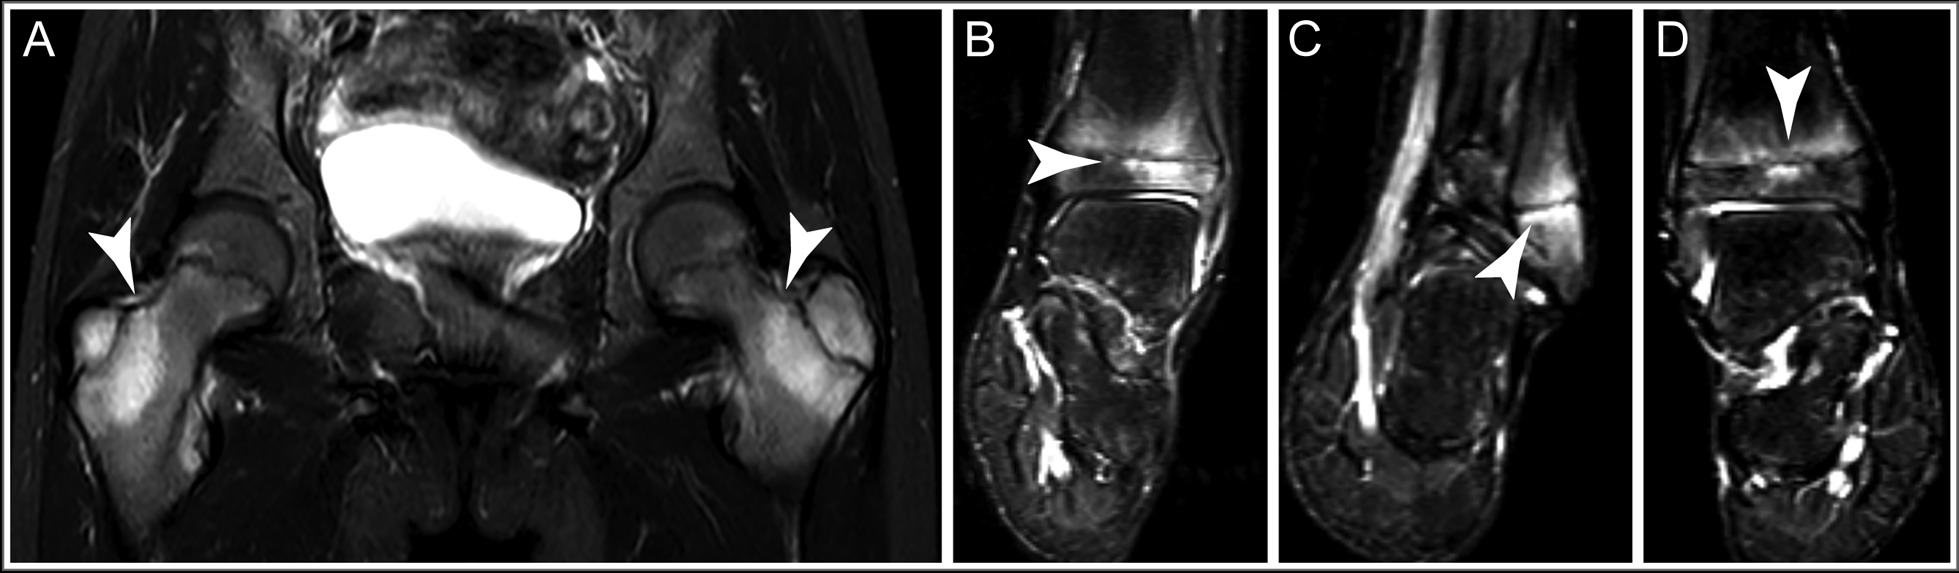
Magnetic resonance imaging of the hips and ankles. Short-tau inversion-recovery (STIR) sequence of the hips and ankles demonstrated widespread osseous inflammation, as indicated by white arrowheads. Bilateral inflammation of the femur (A) was evident along the greater trochanter apophyseal plates. In the left tibia (B) and fibula (C), inflammation likewise paralleled the physes. Inflammation was more widespread (D), involving the majority of the metaphysis and epiphysis. Joint effusions with trace volume were evident at each of these sites
